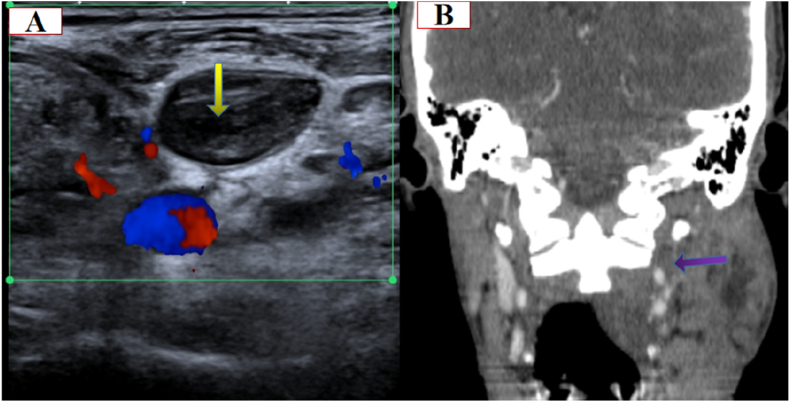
Doppler sonography (A) and contrast-enhanced coronal Computed tomography (B) showed left jugular vein thrombosis.
